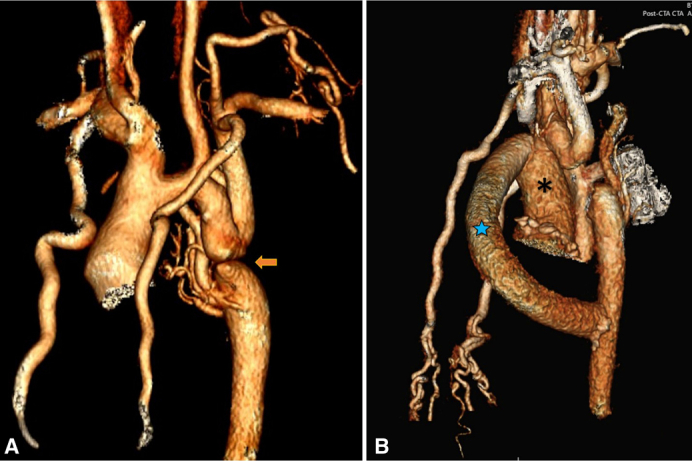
Three-dimensional volume-rendered computed tomography angiography (CTA) reconstructions.
